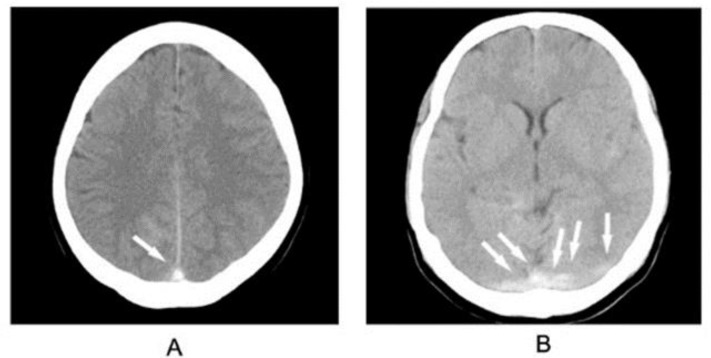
Brain non-enhanced computed tomography: dense triangle sign (A, arrow), dense clot sign (B, arrows
